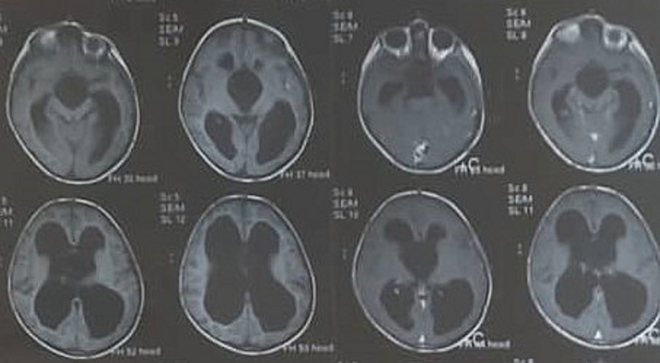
Pre- and post-contrast T1WI sagittal MRI showing large non-enhancing cyst within 3rd ventricle herniated into suprasellar and prepontine cisterns.
